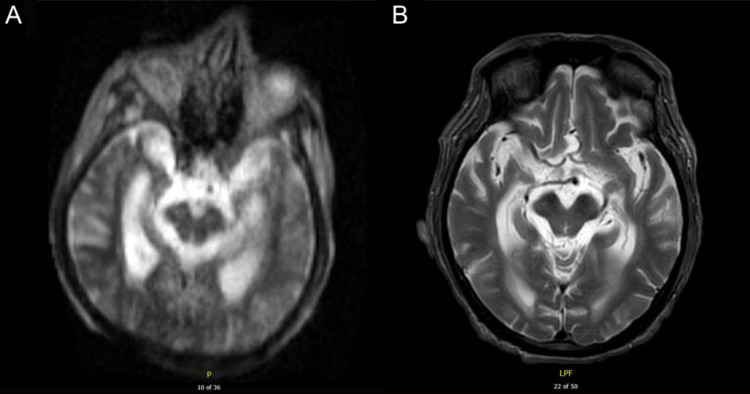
A T2-weighted image from a portable brain scan using a 0.064T magnet without intravenous contrast (A) demonstrates prominent ventricles with patency of the suprasellar and quadrigeminal plate cisterns, which is confirmed on a 3T MRI (B). MRI, magnetic resonance imaging.
